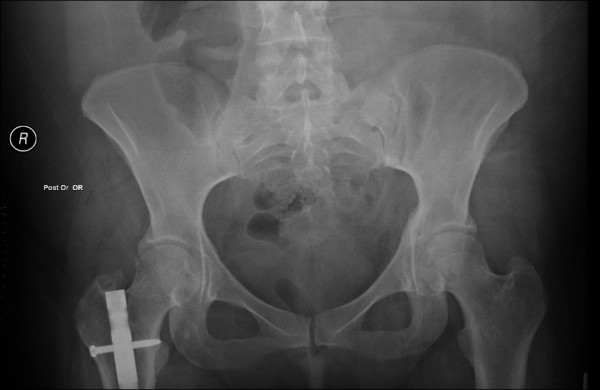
A postoperative pelvic radiograph documents the successful resection of the heterotopic ossification around the right hip.
